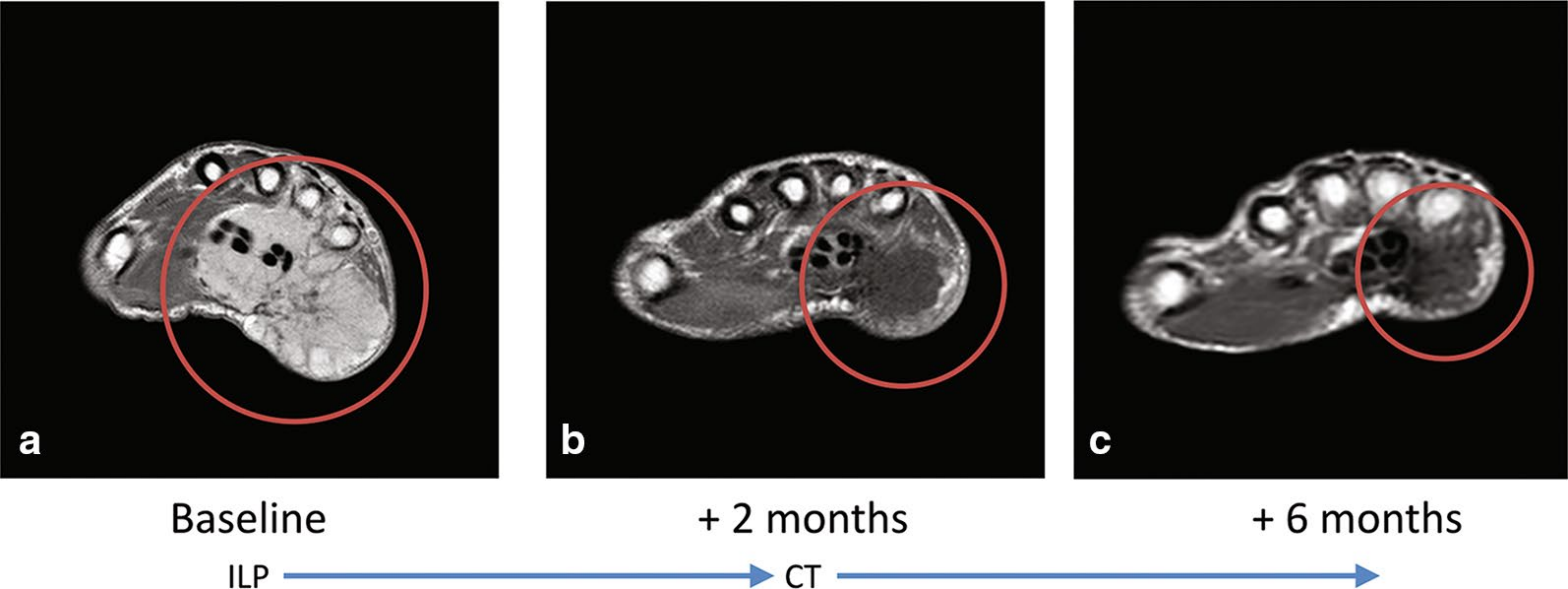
Axial contrast enhanced T1-weighted MRI. Pathologic tissue in the hypothenar eminence of the right hand, involving the flexor tendons and the spaces between 3rd, 4th and 5th metacarpal bones with intense and homogeneous contrast enhancement (a). Progressive size and contrast enhancement reduction after ILP (b), and chemotherapy (c)
